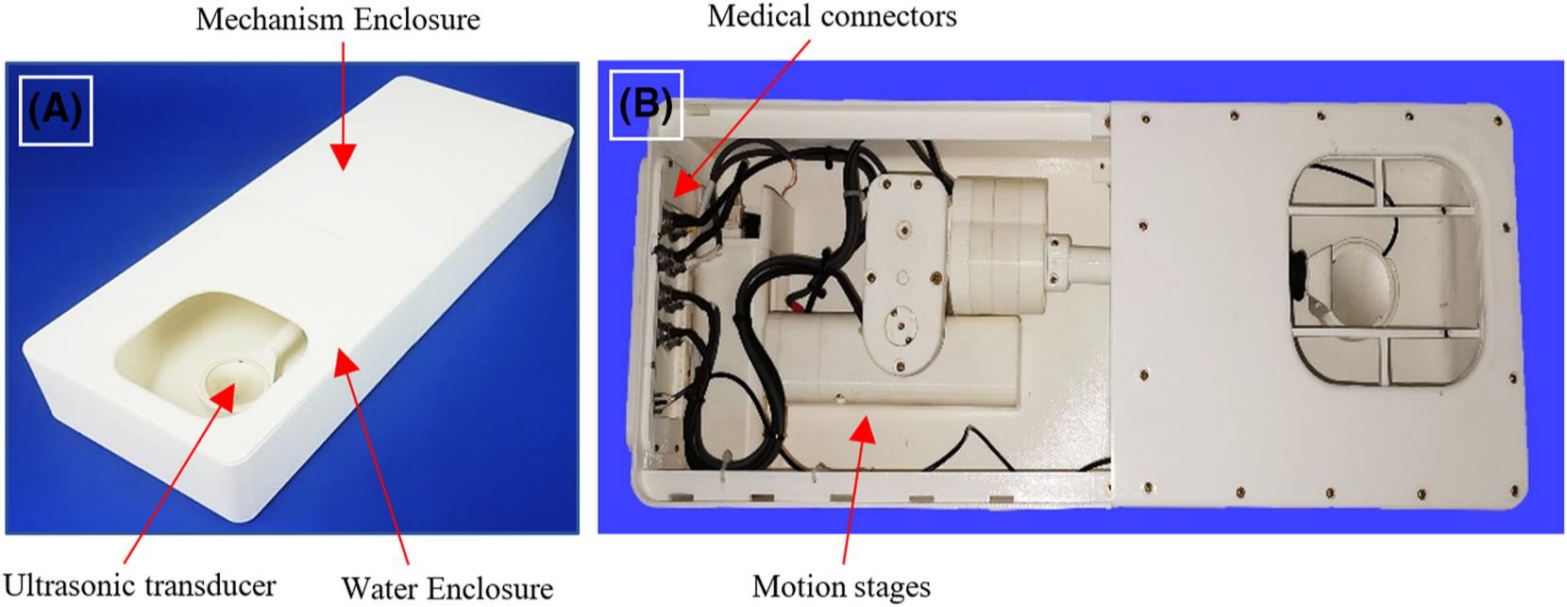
(A) Exterior and (B) interior views of the 4 degrees of freedom (DOF) Magnetic Resonance guided Focussed Ultrasound (MRgFUS) robotic device
